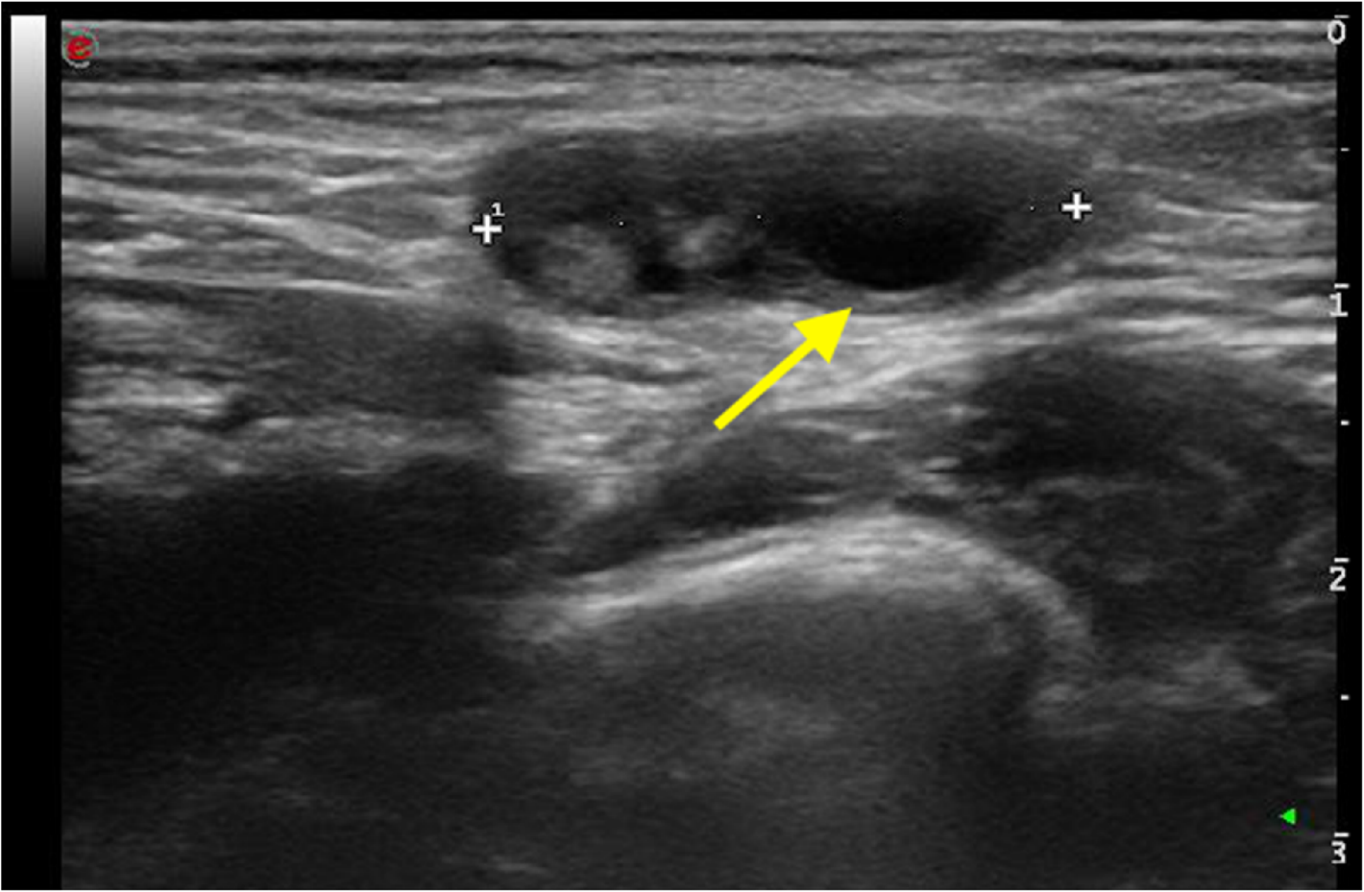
The observation of the follicles within the solid formation (arrow), as well as its tense-elastic texture and the presence of vascularization at Color Doppler, made likely the hypothesis of an annexe
